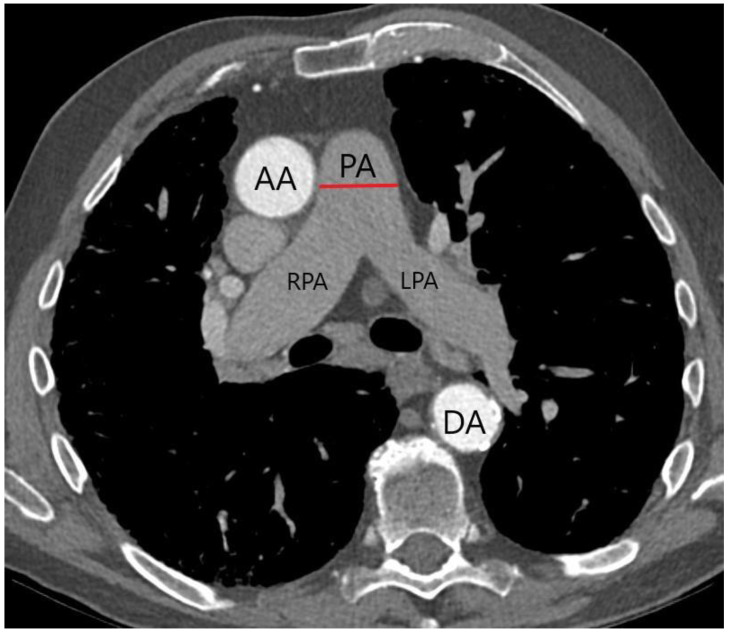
Measurement of PA diameter (red line) on axial CTA. PA = pulmonary artery; RPA = right pulmonary artery; LPA = left pulmonary artery; AA = ascending aorta; DA = descending aorta.
